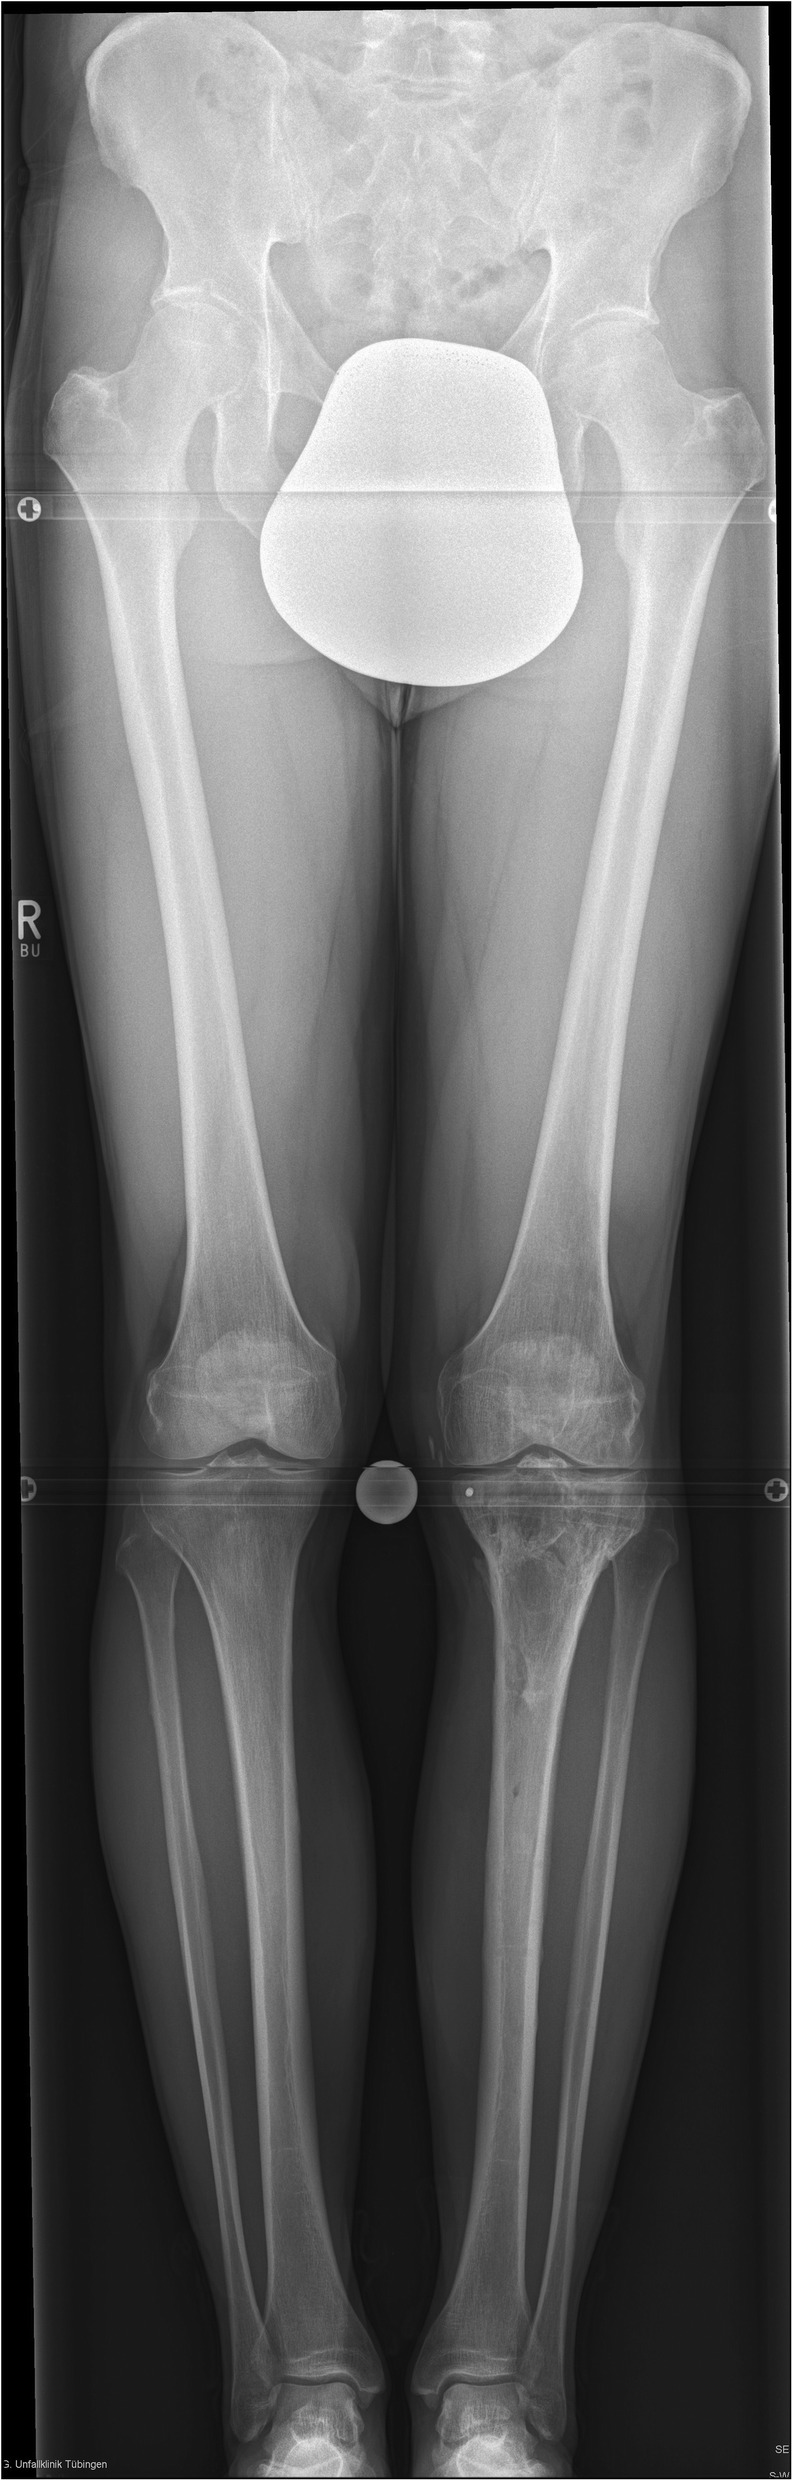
Long standing X-ray showing straight axis with no difference to contra-lateral side
